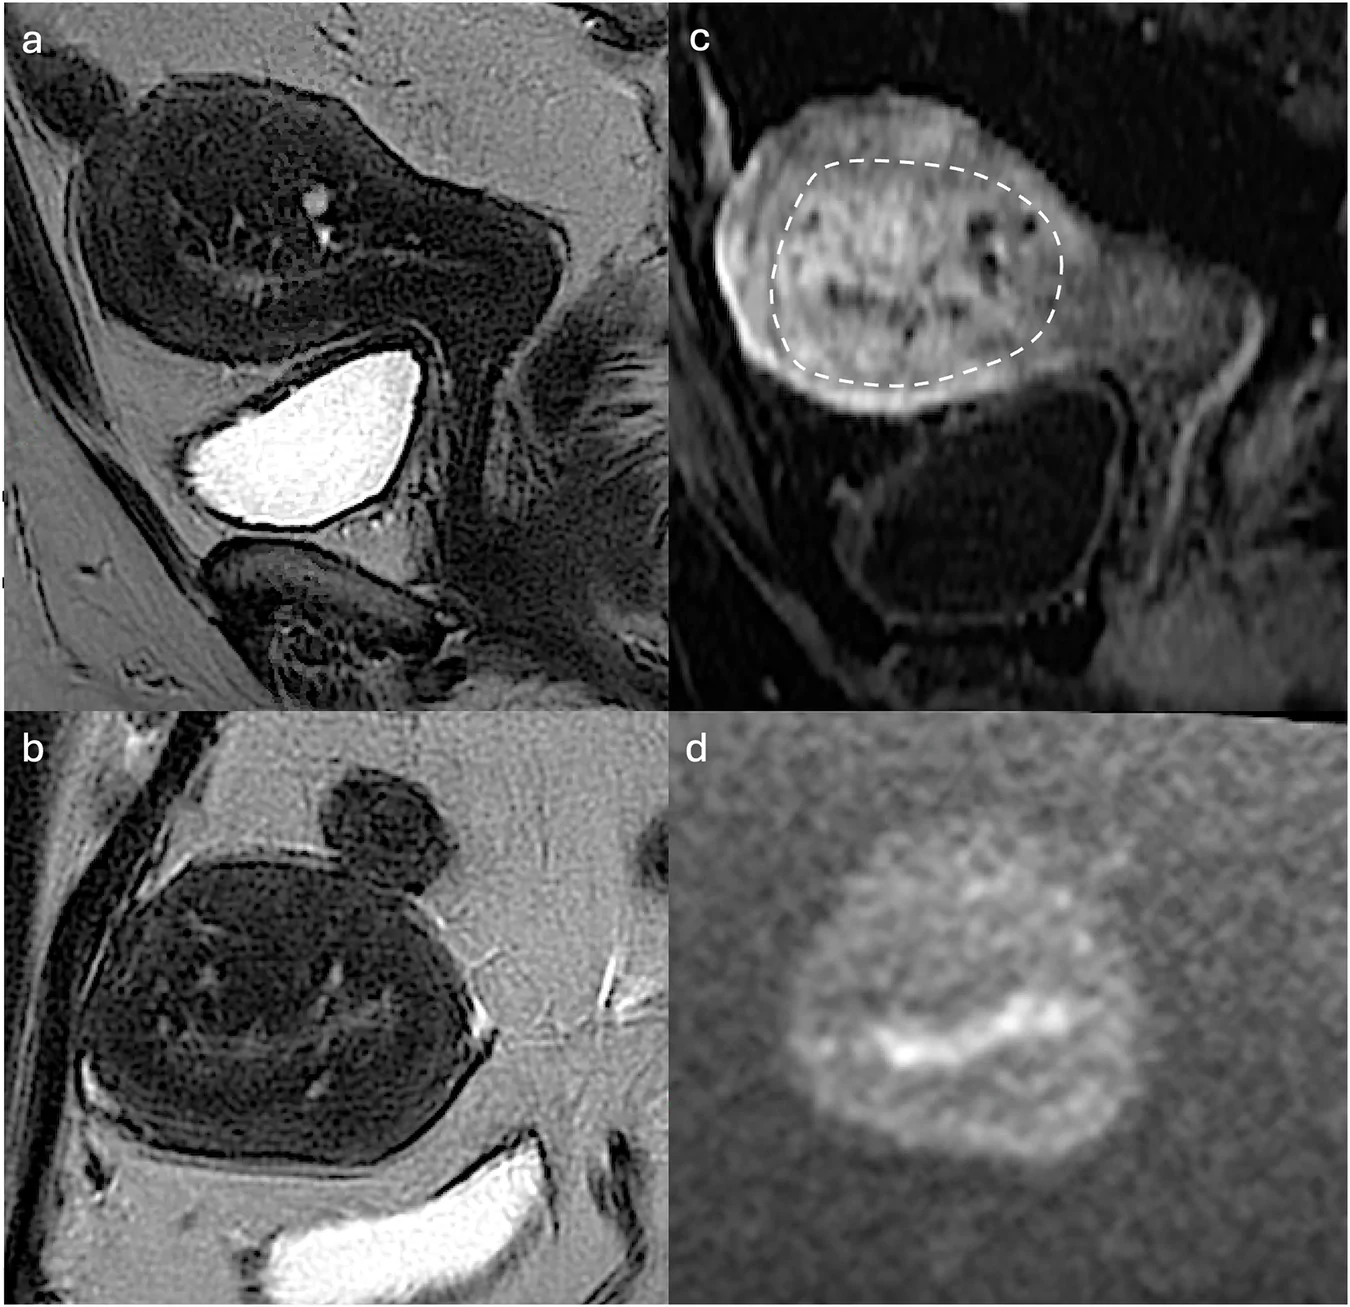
Adenomyosis mimicker: a case of endometrial stromal sarcoma (ESS). a Sagittal T2-weighted sequence; b sagittal postcontrast fat-saturated T1-weighted sequence; c coronal T2-weighted sequence; d coronal DWI sequence. The images show an ill-defined lesion extending into both the endometrial cavity and the uterine wall, with a cystic component. The anterior uterine wall is difficult to distinguish from the lesion, with possible residual normal low-T2 striations and small cysts possibly mimicking adenomyosis. Postcontrast sequence confirms the ill-defined nature (white broken line) of the lesion with high diffusion signal. ESS, endometrial stromal sarcoma
